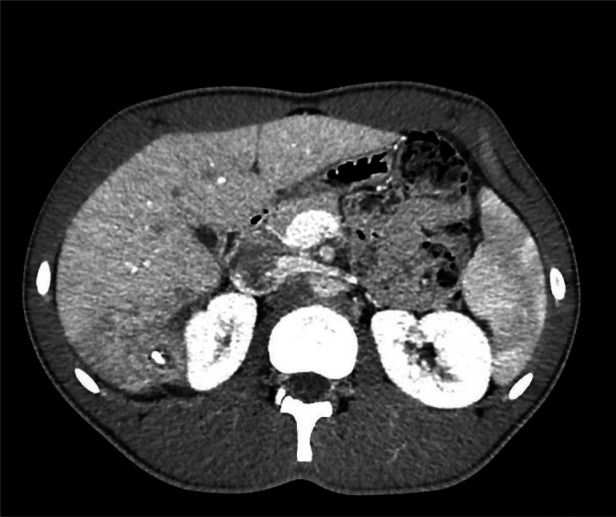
CT scan (after 4 months): Persistent but reduced round collection in S6, showing peripheral enhancement.
